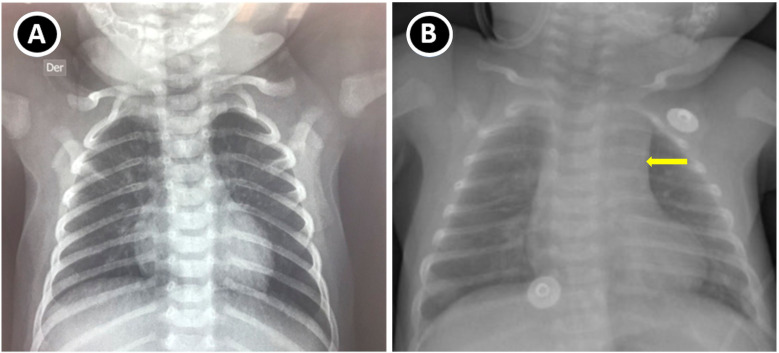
Chest radiography. (A) Chest radiograph of patient P2 showing absence of the thymic shadow, consistent with loss of the anterior mediastinal contour. (B) Normal control with preserved thymic silhouette (yellow arrow).
